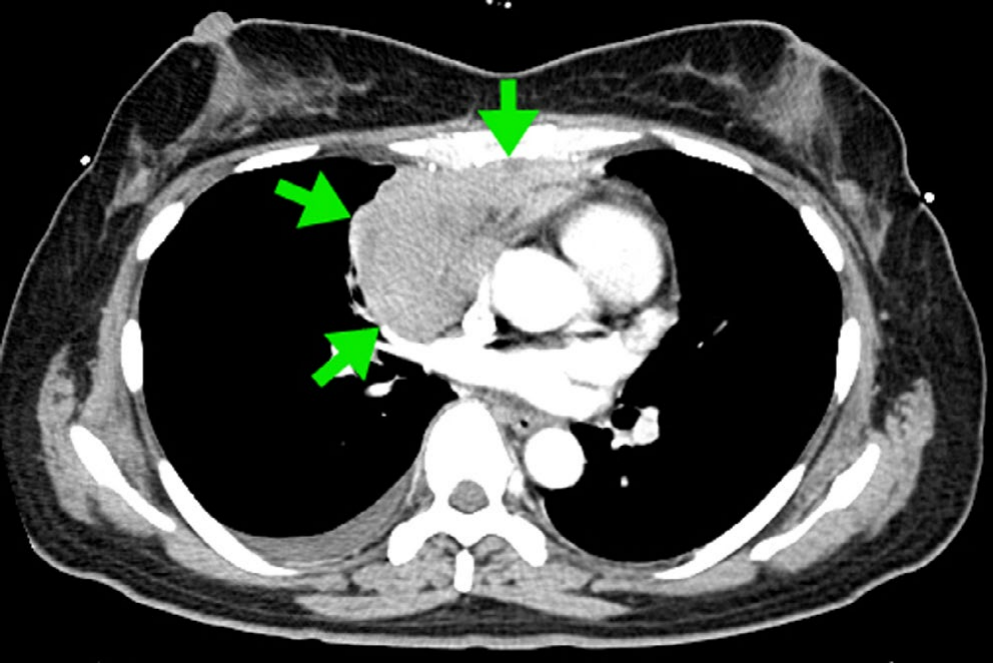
Chest computed tomography showed mediastinal tumor invading anterior chest wall (arrows)
